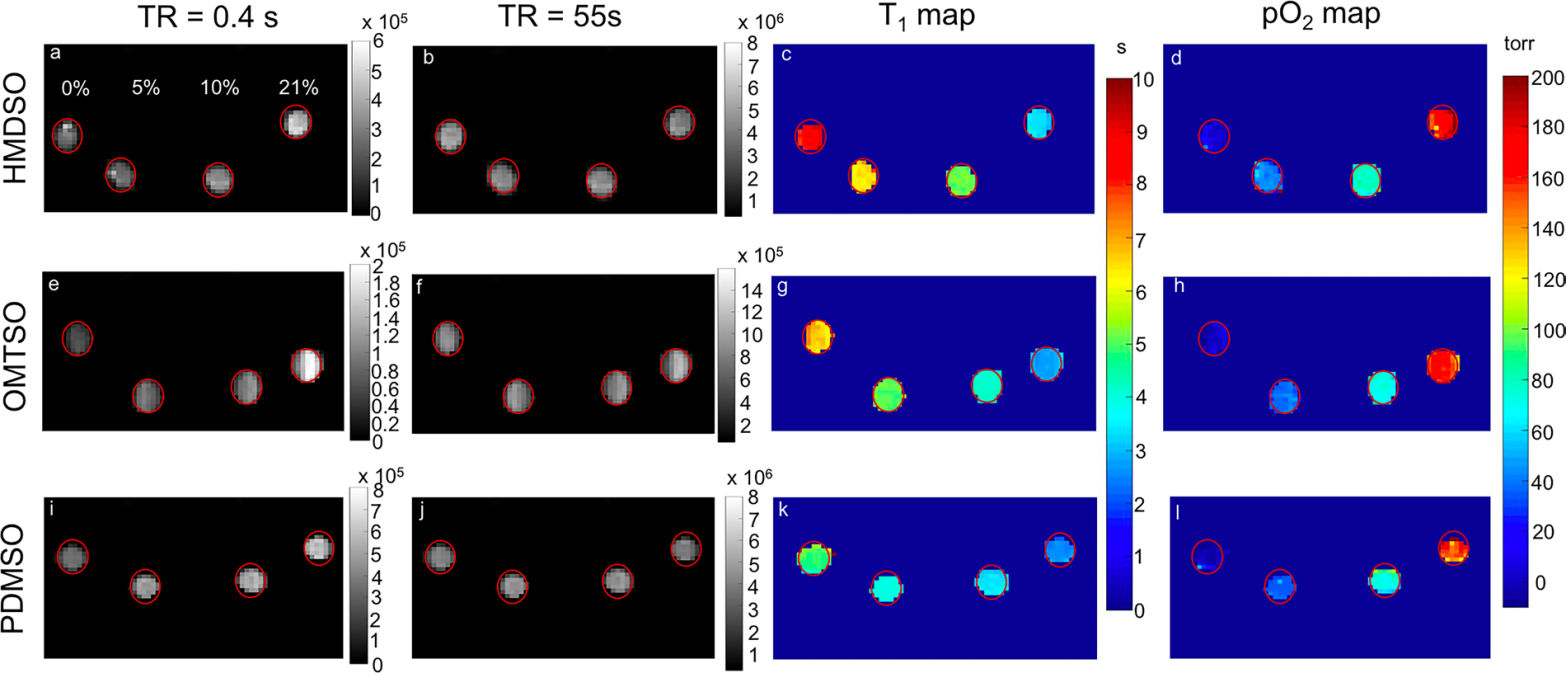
Representative MR images and T_1_ maps from the pO_2_ calibration along with predicted pO_2_ maps for HMDSO (a–d), OMTSO (e–h) and PDMSO (i–l) at 37 °C and 7 T. In each figure the tubes from left to right were bubbled with 0%, 5%, 10% and 21% O_2_ (balance N_2_), respectively. All the images were analyzed using MATLAB R2018b (MathWorks, https://www.mathworks.com/products/matlab.html).
